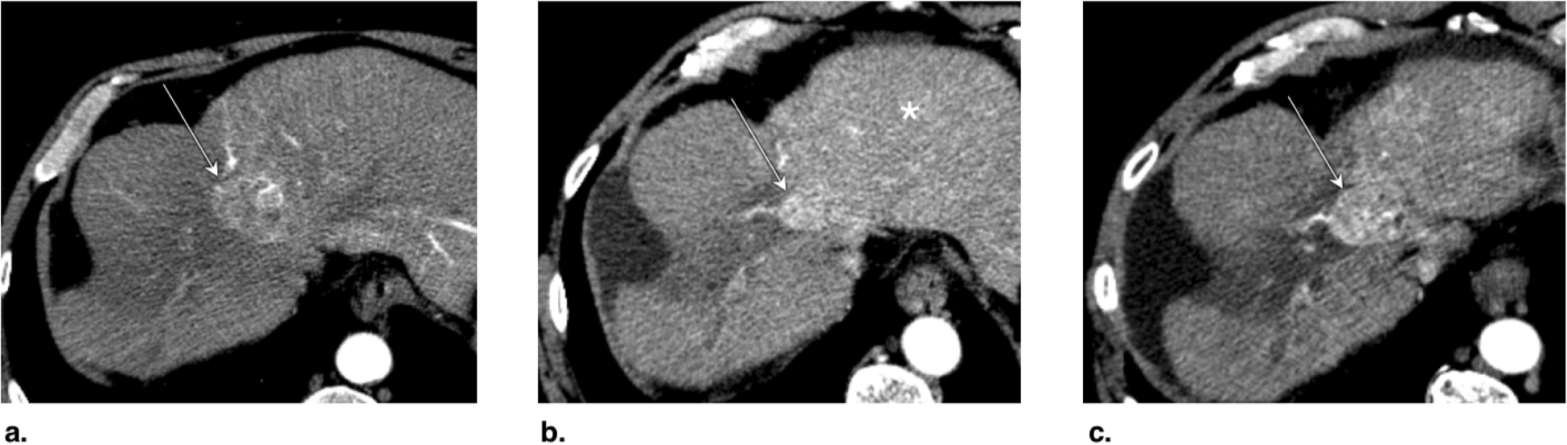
Axial CT in late arterial phase obtained (a) pretreatment: image shows a 4-cm LR-5 observation (arrow) in segment IVa. b 3 months post left lobar transarterial radioembolization (TARE): image shows tumor regression and perfusional changes in left liver (asterisk) related to TARE. c 6 months post TARE: image shows tumor progression (arrow) compatible with recurrence. The treated observation is categorized LR-TR Viable
